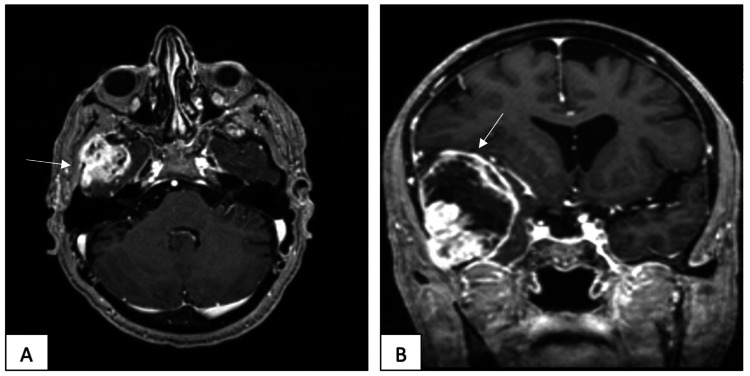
Preoperative MRI The axial (A) and coronal (B) views show a ring-enhancing lesion with central necrosis with midline shaft.
